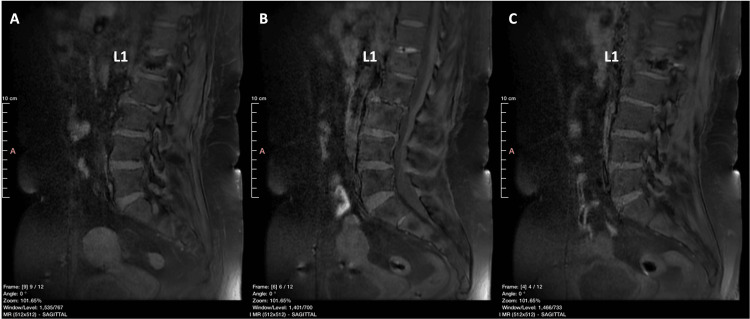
MRI fat suppression T1 weighted image (sagittal view) of the lumbar spine obtained at four months following the two balloons kyphoplasty. No gross signs of a malignant tumor at the L1 vertebra, and/or breaching of the vertebral cortex are apparent. A: left part of the spinal column, B: medial part of the spinal column, C: right part of the spinal column
